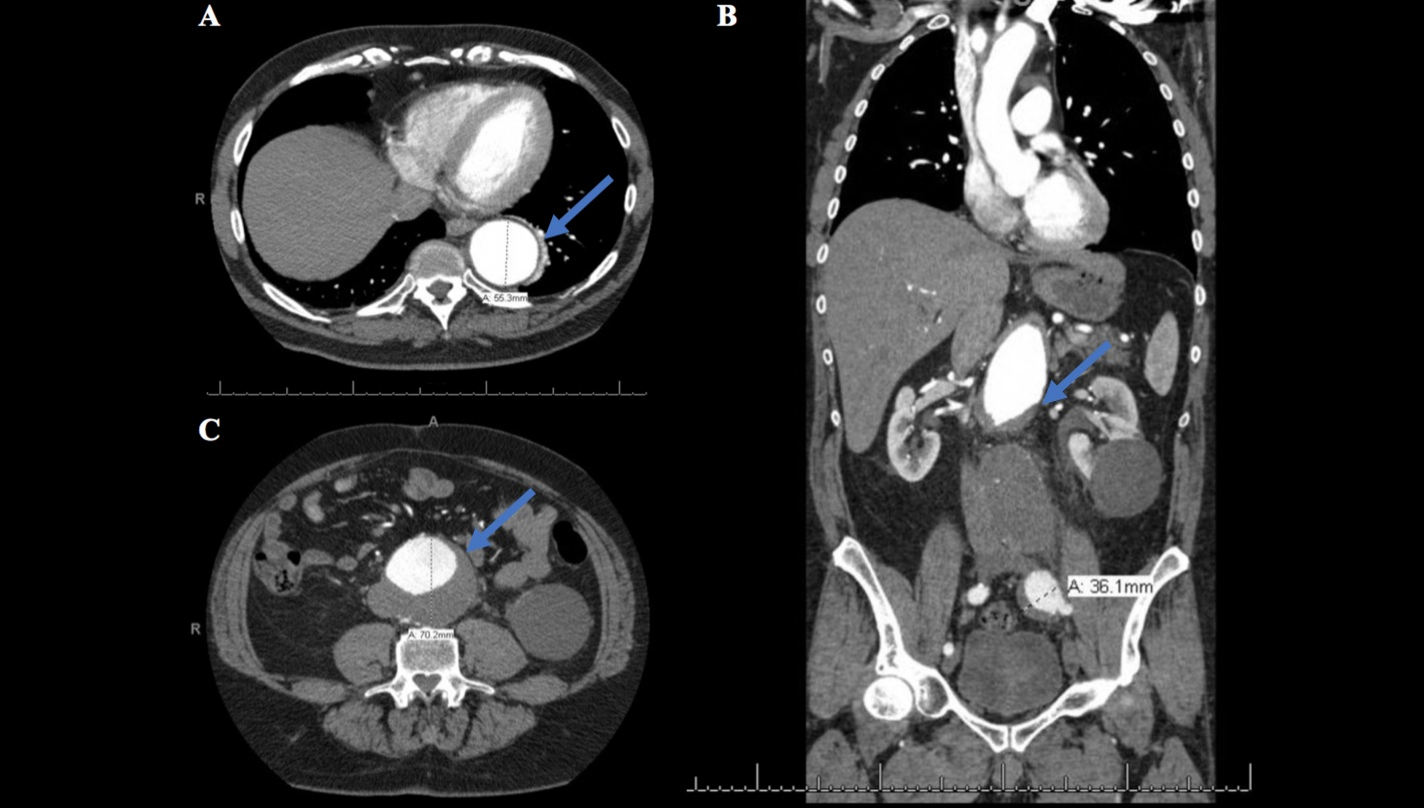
Computed tomography (CT). Transverse and coronal CT imaging of the thorax and abdomen with contrast which demonstrated an acute intramural hematoma extending from the ascending aorta, aortic arch, into the descending thoracic aorta. The aortic root measures up to 3.4 cm. The descending thoracic aorta is dilated and measures up to 5.5 cm. There is dilatation of the intra-abdominal aorta. At the level of the renal arteries, the aorta measures 4.8 cm. Infrarenally, there is an abdominal aortic aneurysm measuring up to 7 cm with eccentric thrombus extending over a length of 10.4 cm extending into the left iliac artery. (A) The descending aorta measured up to 5.5 cm. (B) The left iliac artery measured up to 3.6 cm. (C) The infrarenal aorta measured up to 7 cm.
